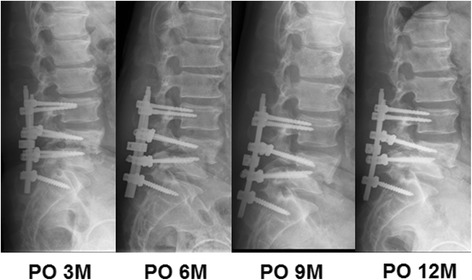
Lateral X-ray of the lumbar spine follow-up from postoperation 3 months, 6 months, 9 months and 12 months. It showed the screws were loosed and bone grafts were absorbed gradually. There is a Charcot’s arthropathy between L4 and L5. PO, postoperation
